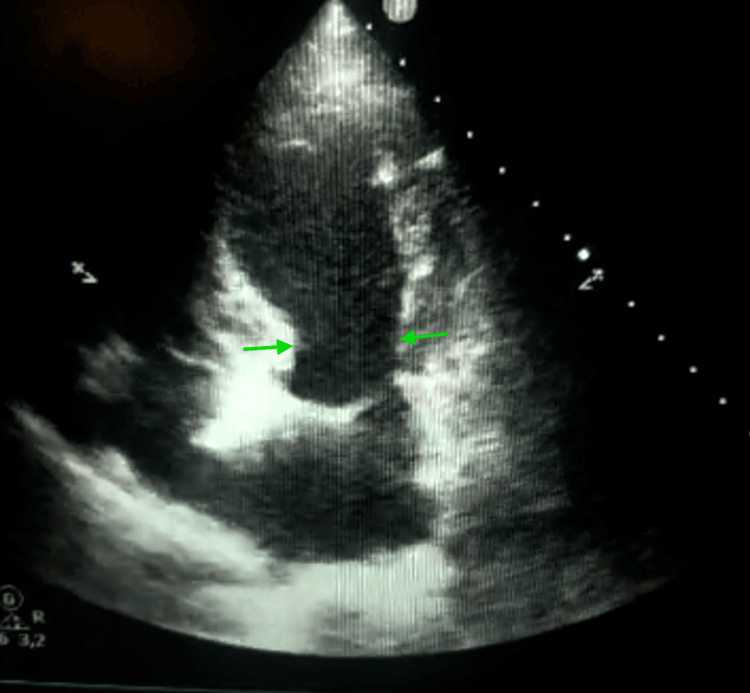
Apical four-chamber transthoracic echocardiographic view obtained on admission. Apical four-chamber transthoracic echocardiographic view obtained at admission, demonstrating severe left ventricular systolic dysfunction with apical akinesia extending to the adjacent mid-ventricular segments, relative basal hypercontractility, and a visually estimated left ventricular ejection fraction of 20%-25%. The arrows indicate the hypercontractile basal segments.
